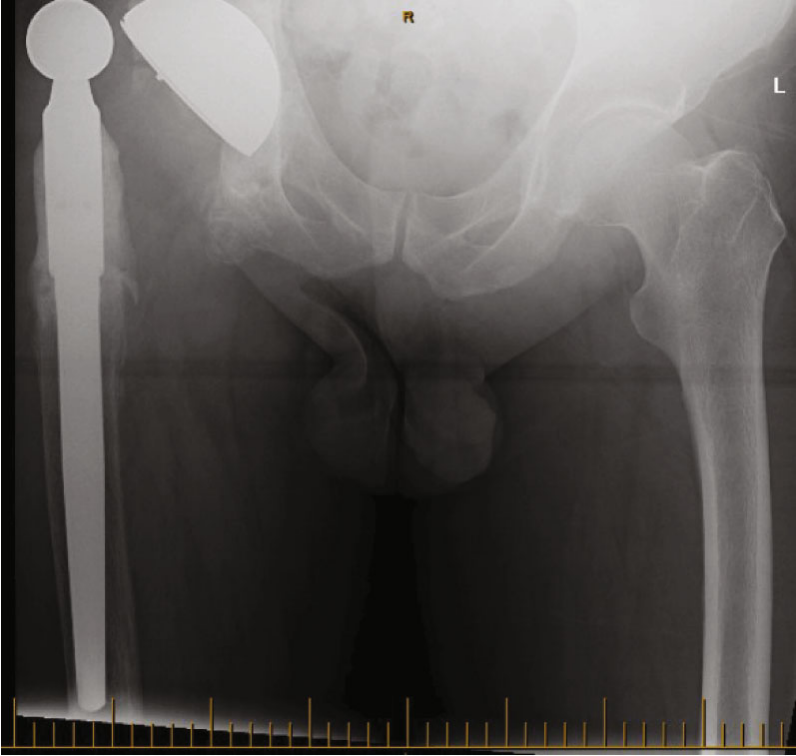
Pelvic X-ray 2017 showing dislocation of the revision prosthesis.
